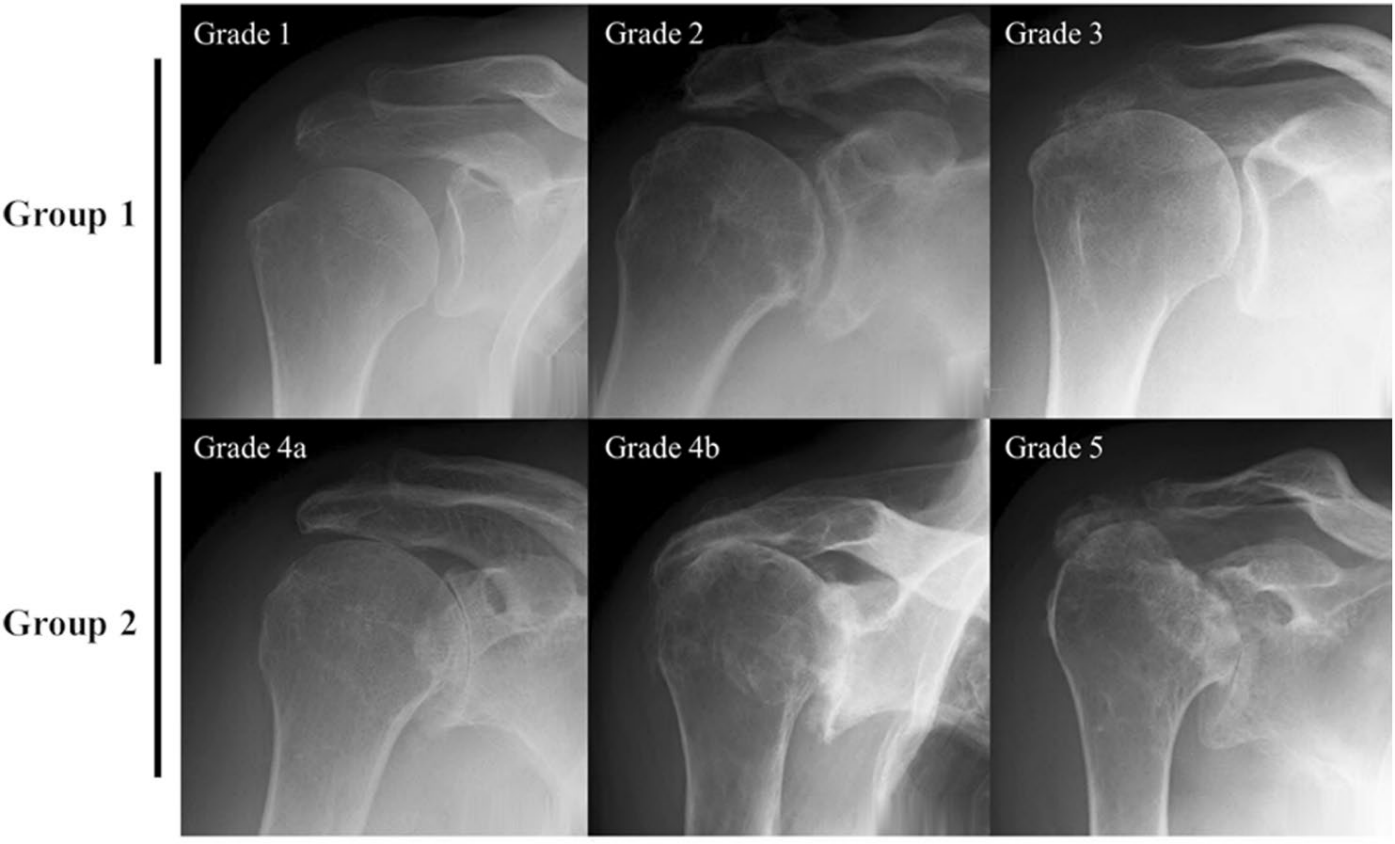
Radiographic images of Hamada classification grades
